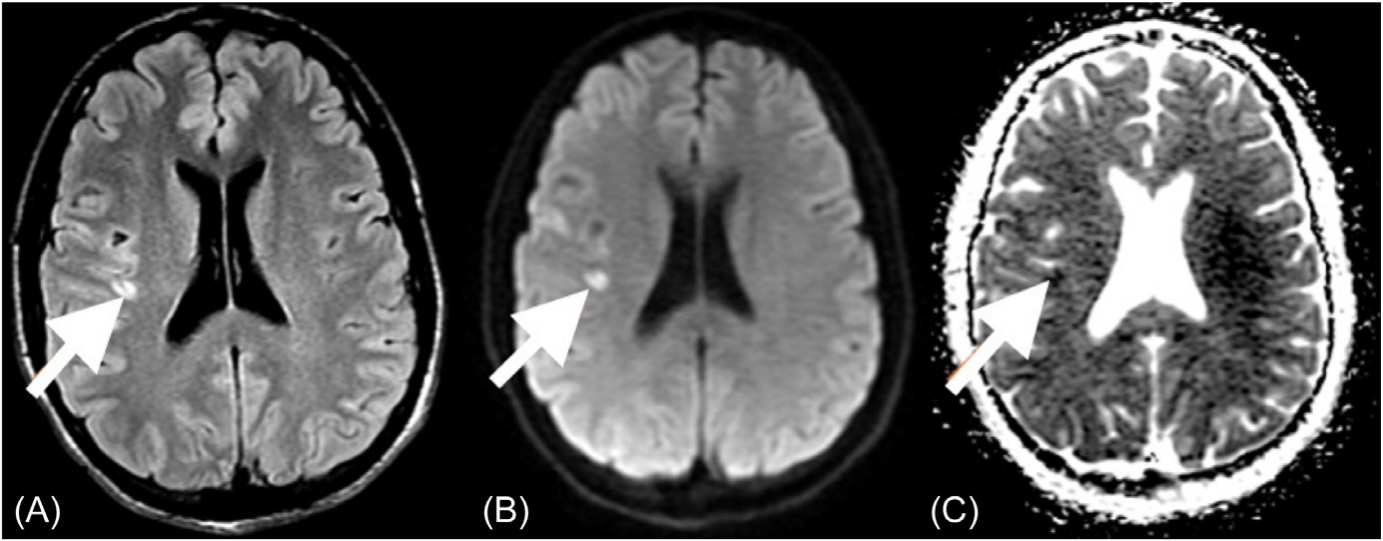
Non-contrast brain magnetic resonance imaging demonstrates a hyperintense lesion on the axial T2- weighted image in the posterior right insular cortex (A) with restricted diffusion as demonstrated by focus of hyperintense signal on the diffusion weighted image (B), and a focus of hypointense signal on the apparent diffusion coefficient map (C), consistent with an acute infarct. Similar lesions were also identified in the anterior right frontal lobe and anterior aspect of the right parietal lobe.
